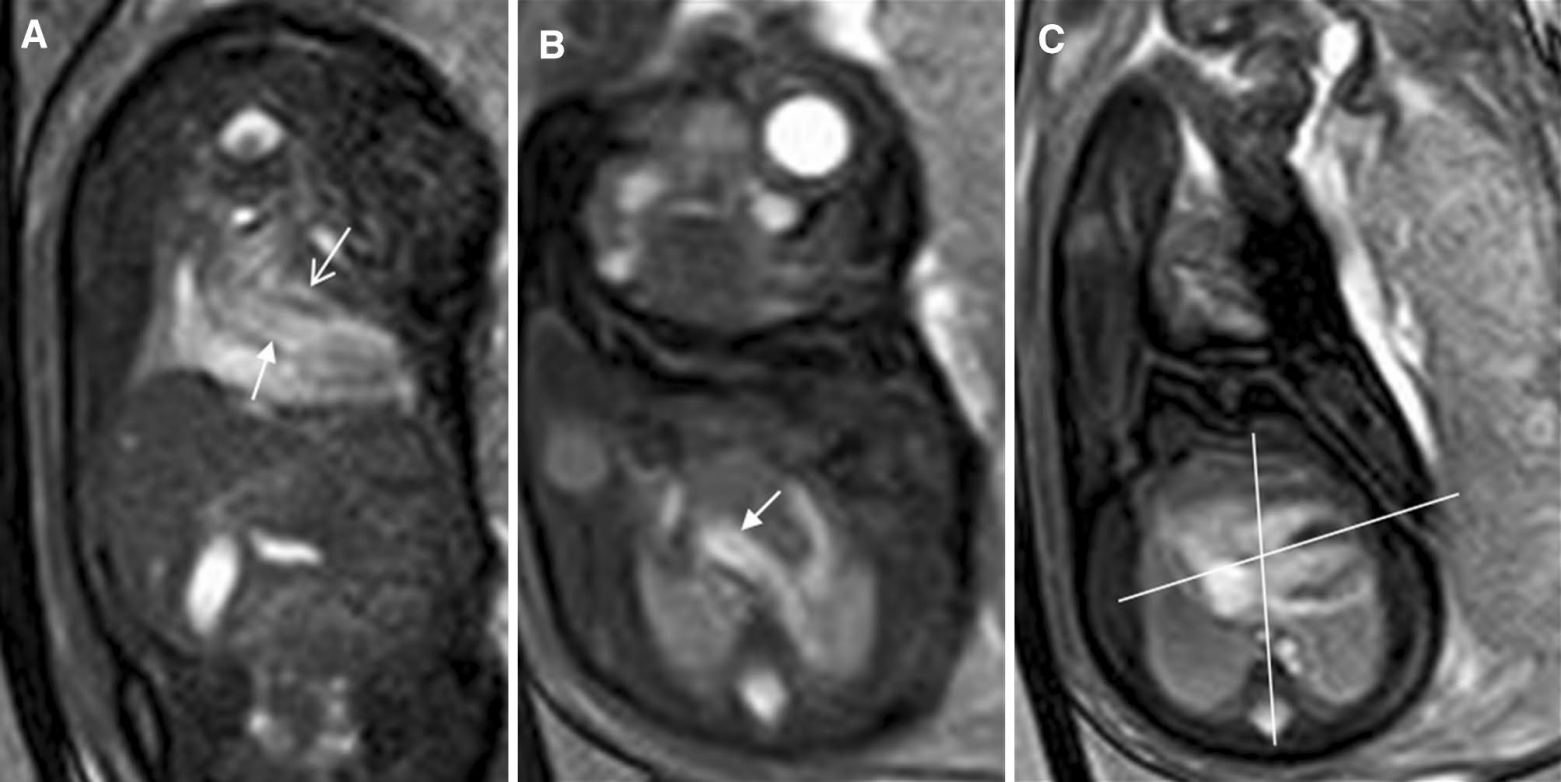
A 28-week-old foetus with a TOF referred for a foetal cardiac MRI because of oligohydramnios. Foetal CMR SSFP coronary and axial view images showing the oligohydramnios and stenosis of the right ventricle outflow tract (open arrow in A), the enlarged ascending aorta (arrow in A), the aortic arch (arrow in B), and the turning right cardiac axis (the angle between the line through the foetal spine and sternum and the interventricular septum in C).
